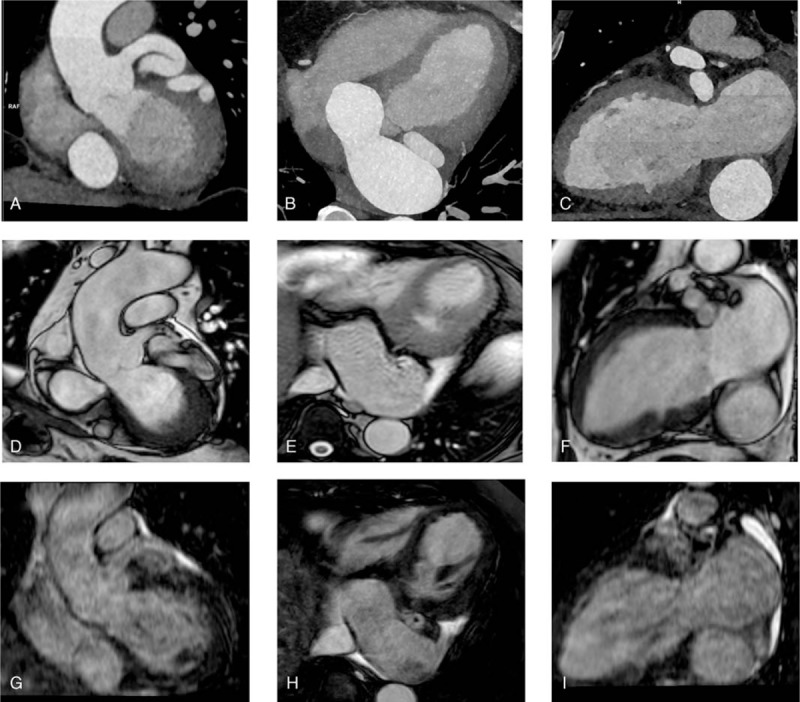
(A-D-G) Cardiac computed tomography (CCT) multiplanar reformation (MPR), cardiac magnetic resonance (CMR) three-chamber view and CMR SSFP MPR representing the origin of the fistula. (B-E-H) CCT MPR, CMR four-chamber view and CMR SSFP MPR showing the enlarged coronary sinus. (C-F-I) CCT MPR, CMR two-chamber view and CMR SSFP MPR where proximal and distal left circumflex coronary artery and the coronary sinus appear both dilated.
